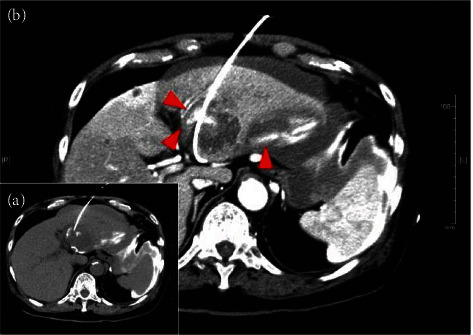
Plain-computed tomography (a) and contrast-enhanced computed tomography (b) after percutaneous transhepatic abscess drainage (PTAD). Extravasation of contrast media and hematoma was observed around the liver and spleen (b, arrowhead).
